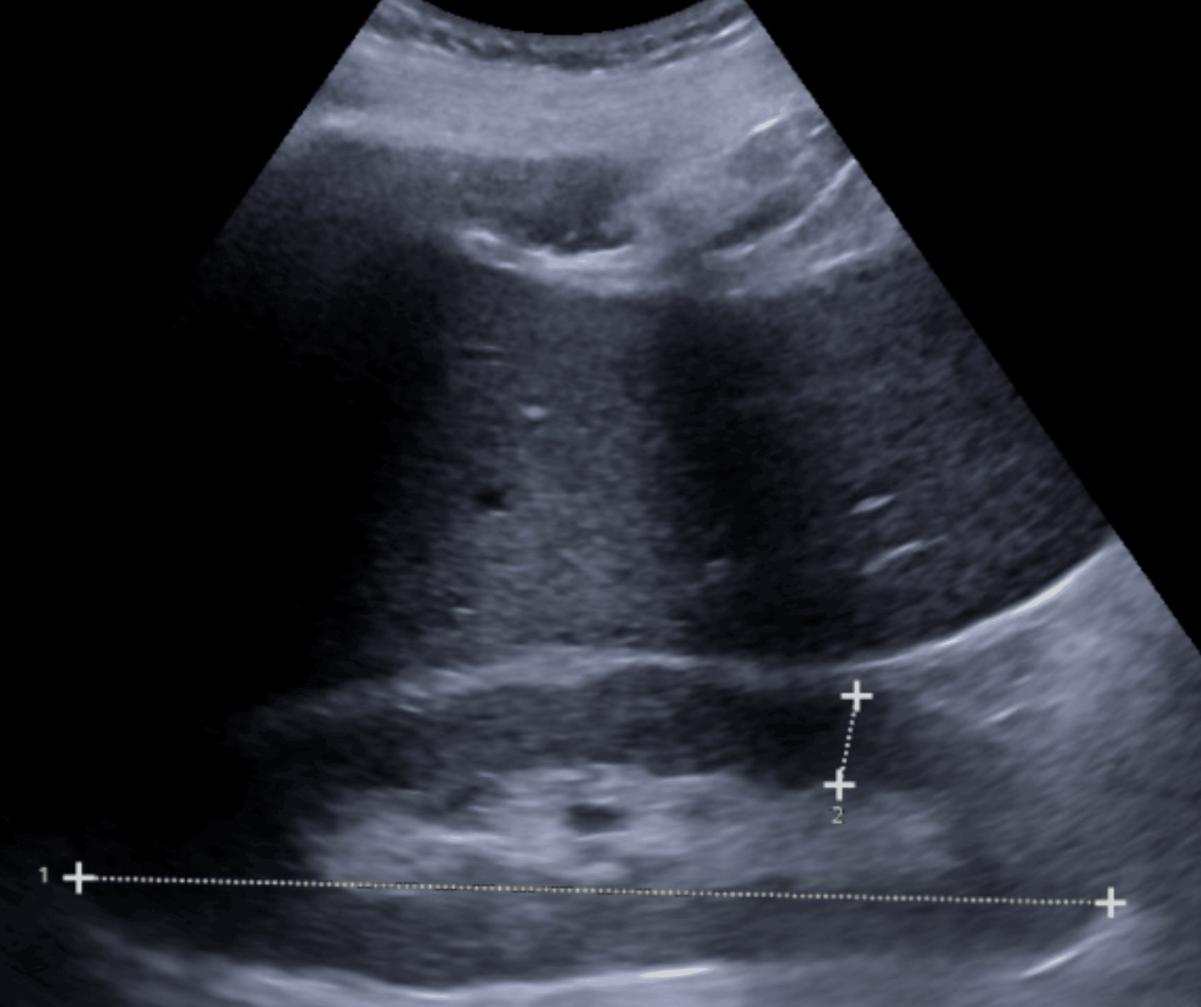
Renal ultrasound of the right kidney Line number 1 shows the right kidney measuring 15.67 cm in length (reference range: 10-14 cm). Line number 2 shows the right kidney cortical thickness of 1.38 cm (reference range: 0.7-1.1 cm).
